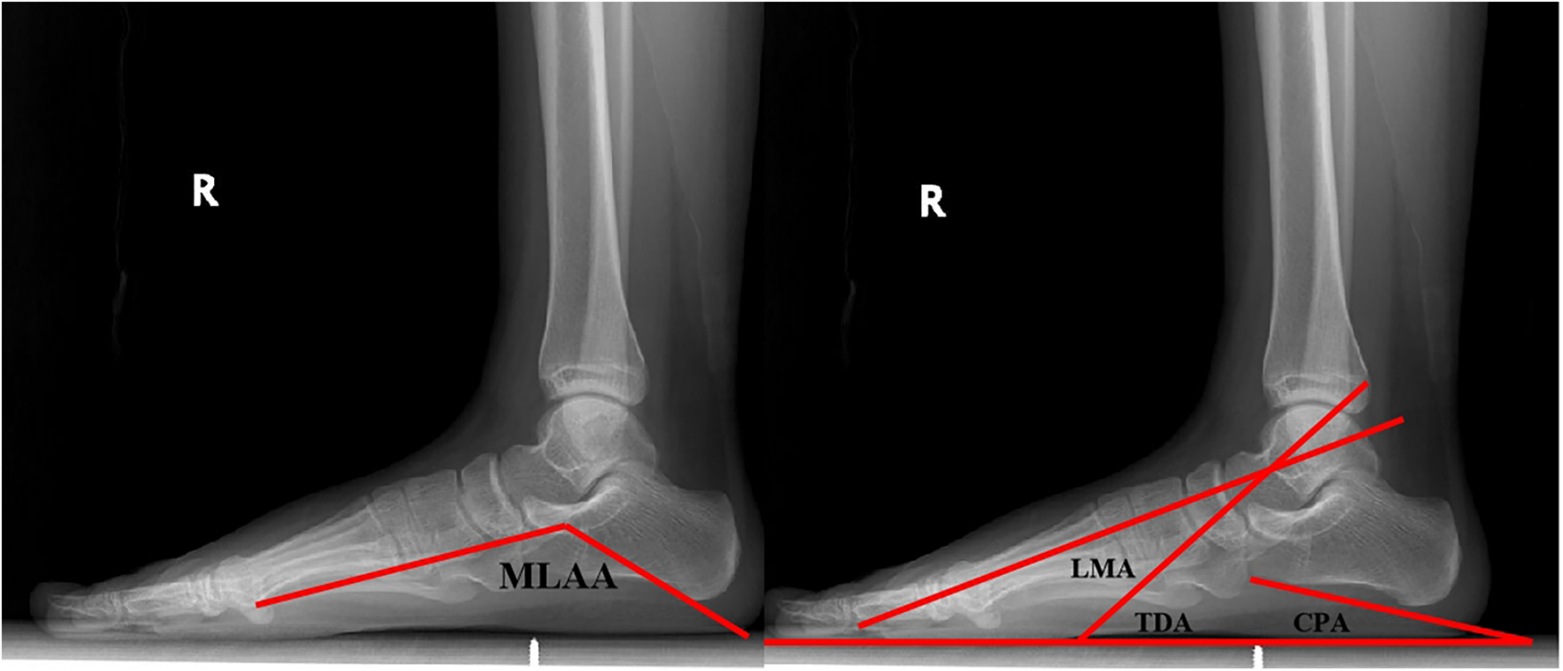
Lateral view of radiograph, demonstrating the measured angles. CPA formed by a line connecting the two most inferior points on the calcaneus and another line parallel to the ground. LMA formed by the axis of the first metatarsal and the longitudinal axis of the talus. TDA formed by a line parallel to the ground and the longitudinal axis of the talus. MLAA formed by a line connecting the most inferior points on the calcaneus and the caput tali and another line connecting the most inferior points on the head of the first metatarsal and the caput tali.
